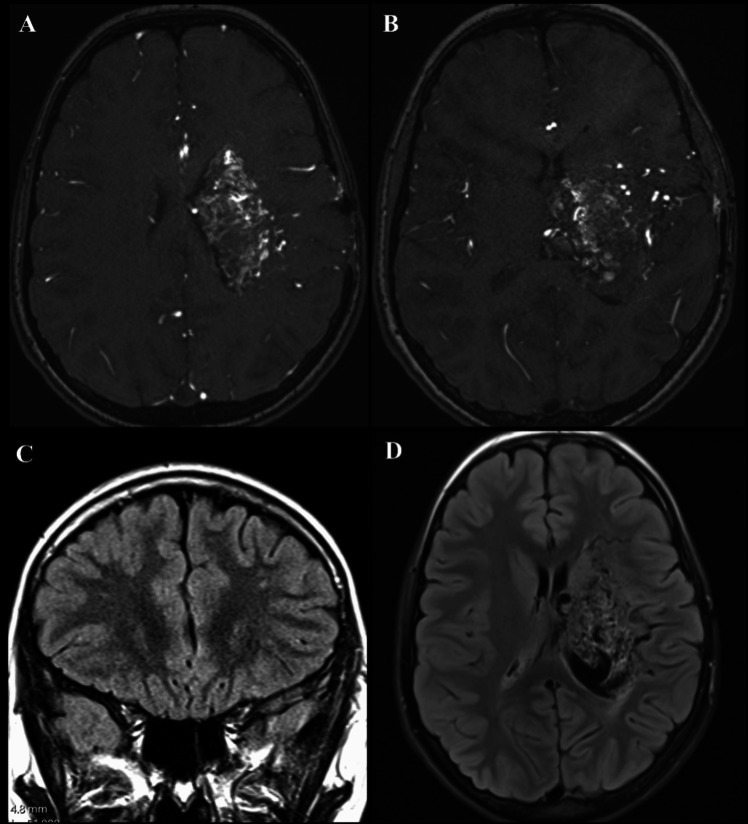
A, B Postoperative MRI at 6 months showing stable bAVM appearances and new cortical supply from the STA graft over the cortical surface. C, D Postoperative FLAIR MRI sequence showing reduction in the Ivy sign within the sulci
